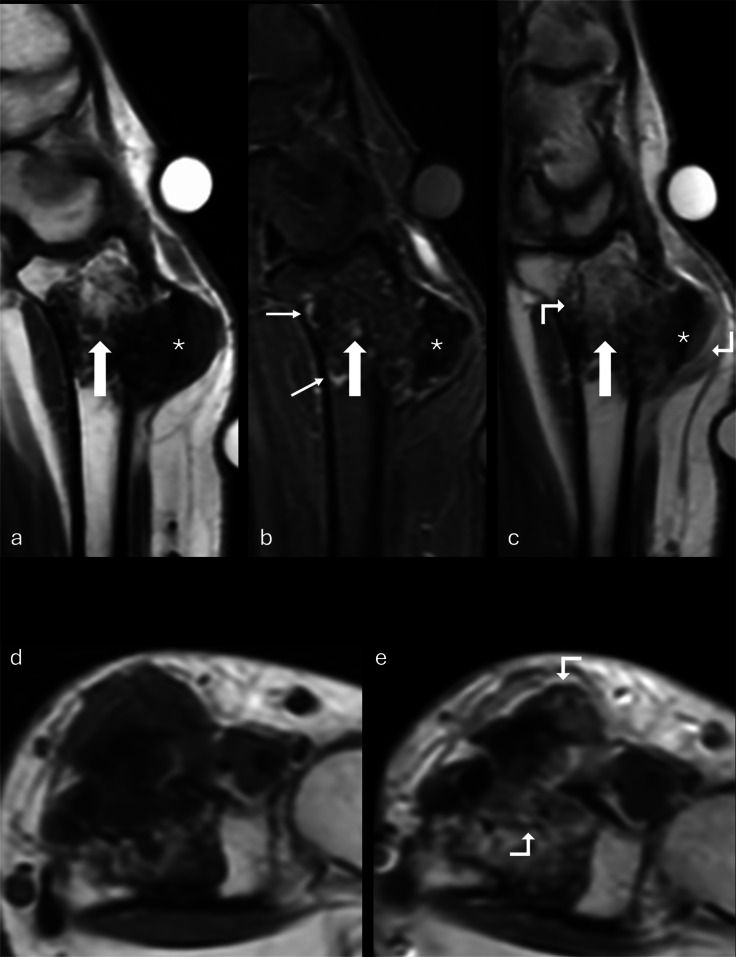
MR images of the right wrist mass. T1-weighted (a), STIR (b), and T1-weighted post-contrast (c) sagittal images; T1-weighted pre- (d) and post-contrast (e) axial images. Relatively homogeneous low signal intensity is seen in the ossified exophytic mass (asterisk, a–c); lobular intramedullary tumor reveals patchy low signal (block arrow), merging with fatty marrow and poorly distinguished on STIR images (b). Thin peripheral high signal intensity is seen (thin arrows, b). Mild enhancement of the intramedullary and predominantly the superficial aspect of the protuberant mass is seen (bent arrows, c, e)
